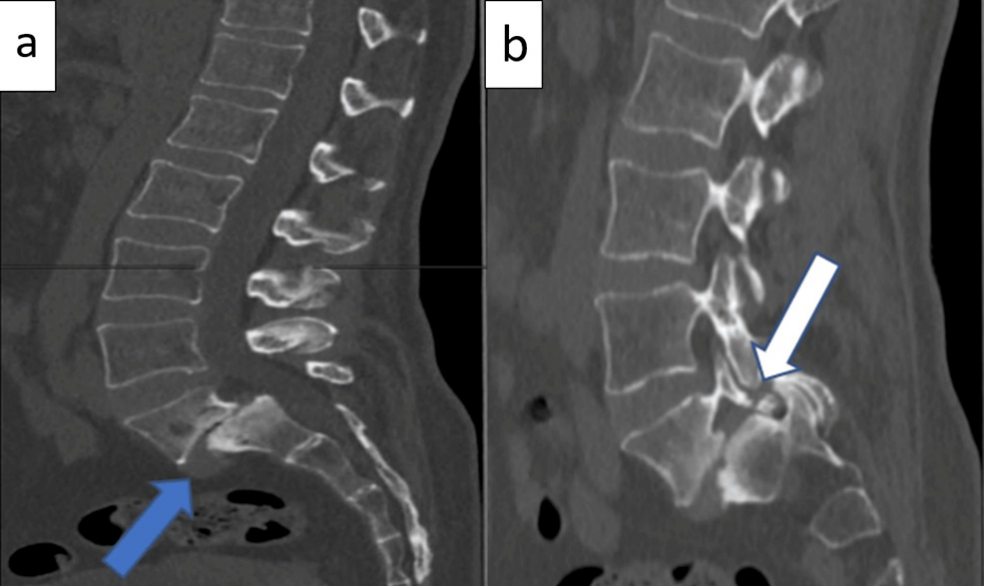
Preoperative sagittal cut CT scan images. a: shows grade 3 spondylolisthesis of L5 (blue arrow) and complete loss of the L5-S1 disc space with reactive sclerosis of the endplates. b: shows spondylolysis of L5 (white arrow).
